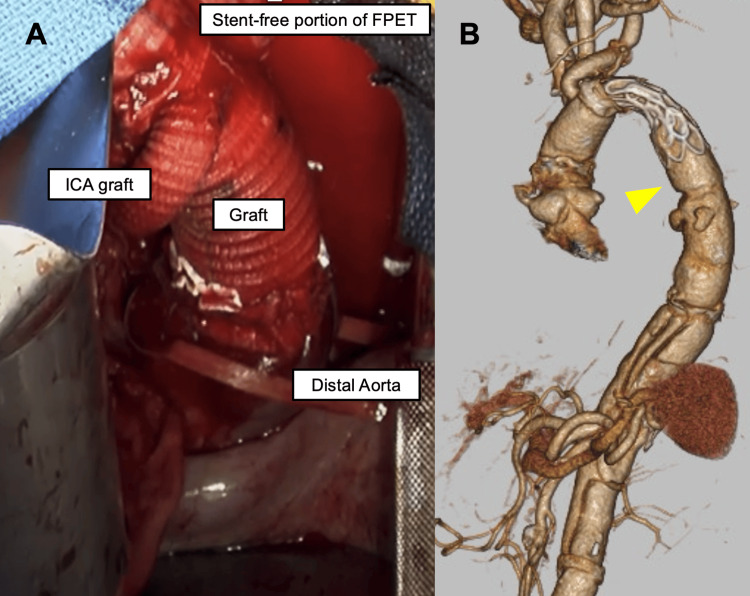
Final result after reconstruction with two-stage surgery A. Intraoperative findings during the second stage left thoracotomy. B. 3D CT image showing the entire aorta after reconstruction. The stent-free portion below the stent frame is the distal end of the FROZENIX Partial ET graft (yellow arrow) CT: computed tomography; FPET: FROZENIX Partial ET; ICA: intercostal artery
